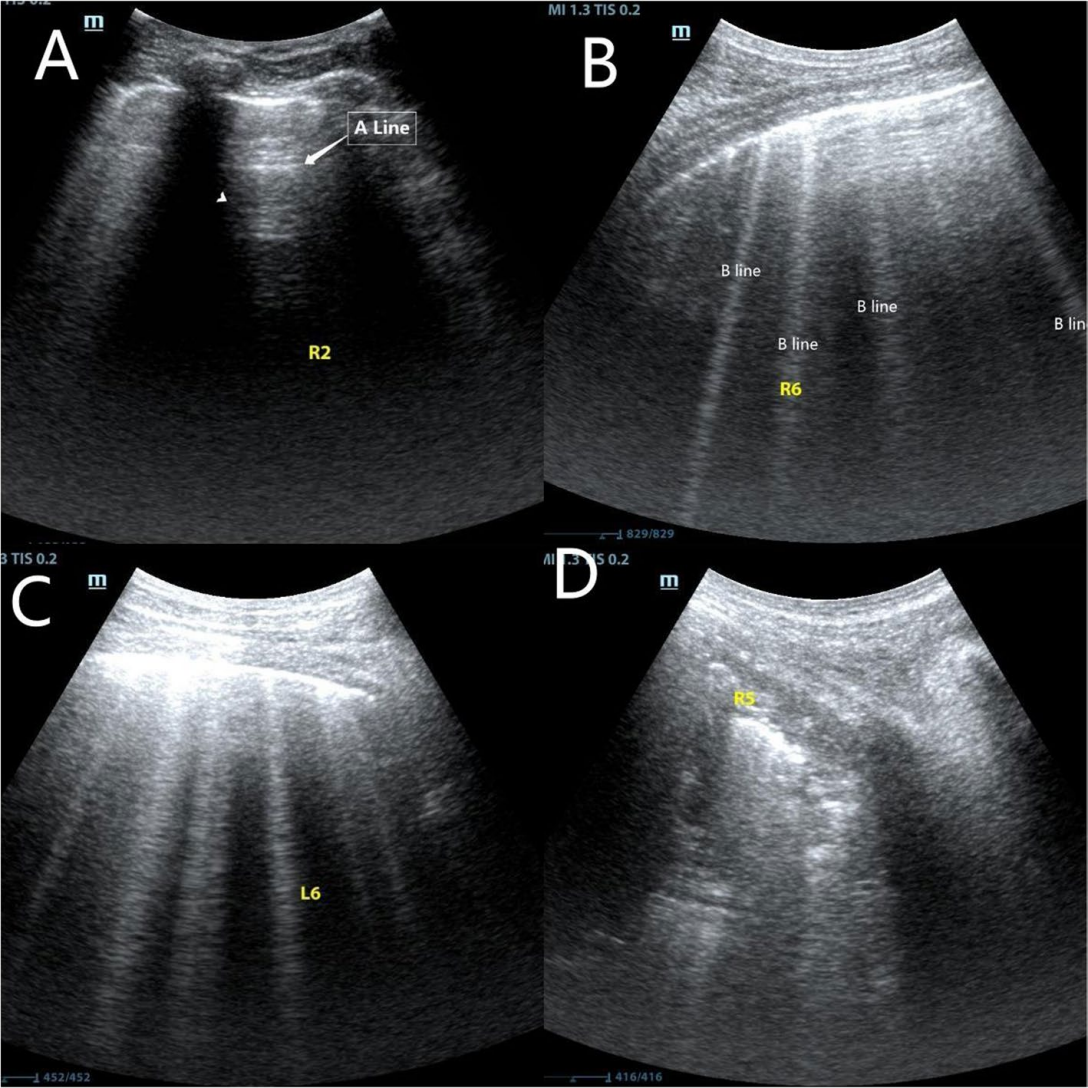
Lung ultrasound scores determined by the number of B lines and subpleural consolidation. A Normal aeration with 0–2 B lines, score = 0, B a small loss of aeration with ≥ 3 B lines, score = 1, C a moderate loss of aeration with multiple coalescent B lines or small subpleural consolidation, score = 2, and D a severe loss of aeration with consolidation or large subpleural consolidation, score = 3. (The yellow letters in the picture are the markings made in the trial. R and L represent the right and left hemithorax, and the numbers represent the subdivisions.)
